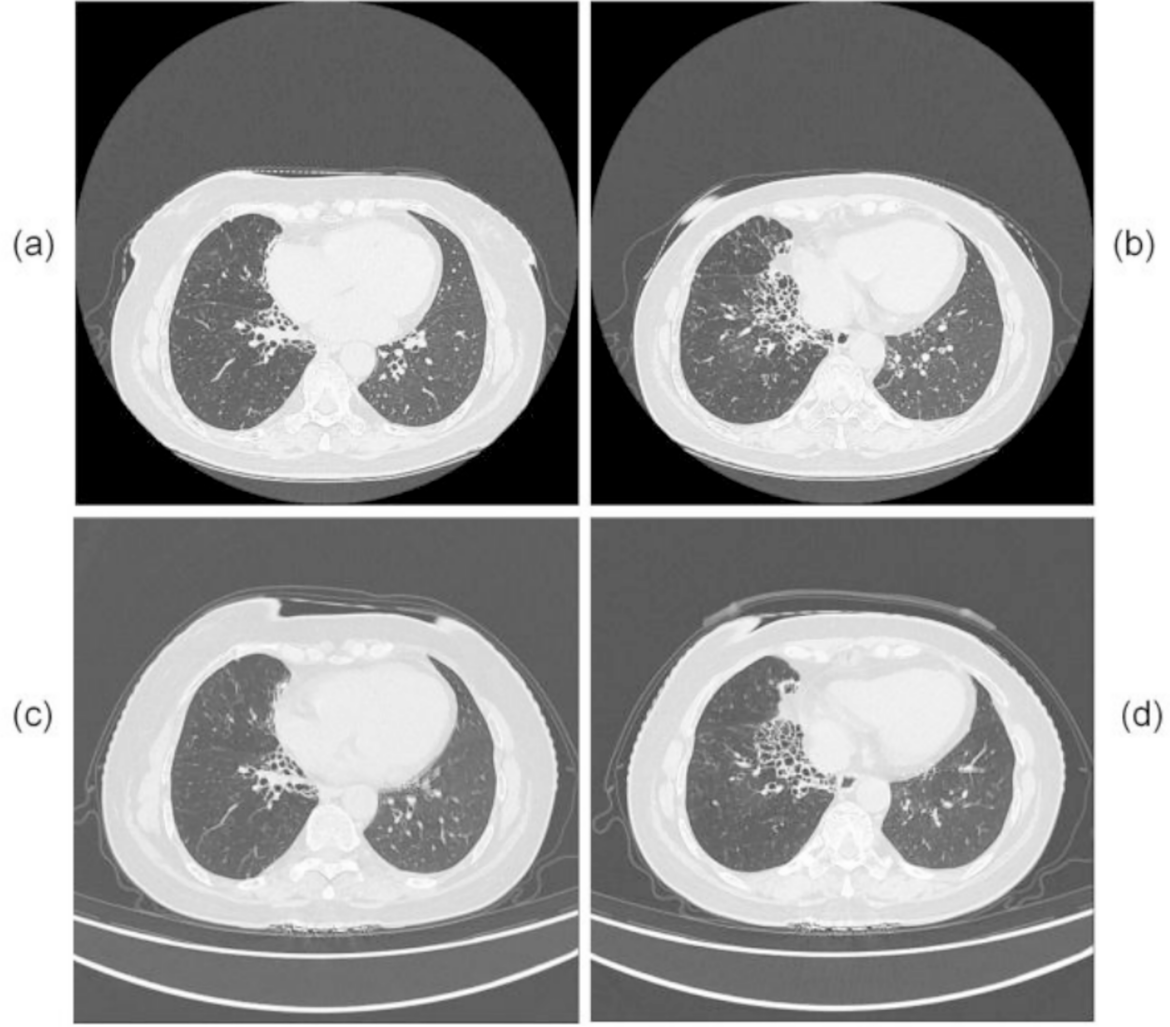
Chest CT of Case 3. After 2 months of treatment: (a,b) multiple nodular and cystic lucencies are seen in both lungs, accompanied by bronchiectasis. The lesions are extensively distributed. Six months after the completion of anti-infection therapy: (c,d) a reduction in bilateral reticular opacities is noted. The morphology of bronchiectasis has stabilized, with pulmonary consolidations and ground-glass opacities showing signs of subsiding, though residual structural alterations persist.
